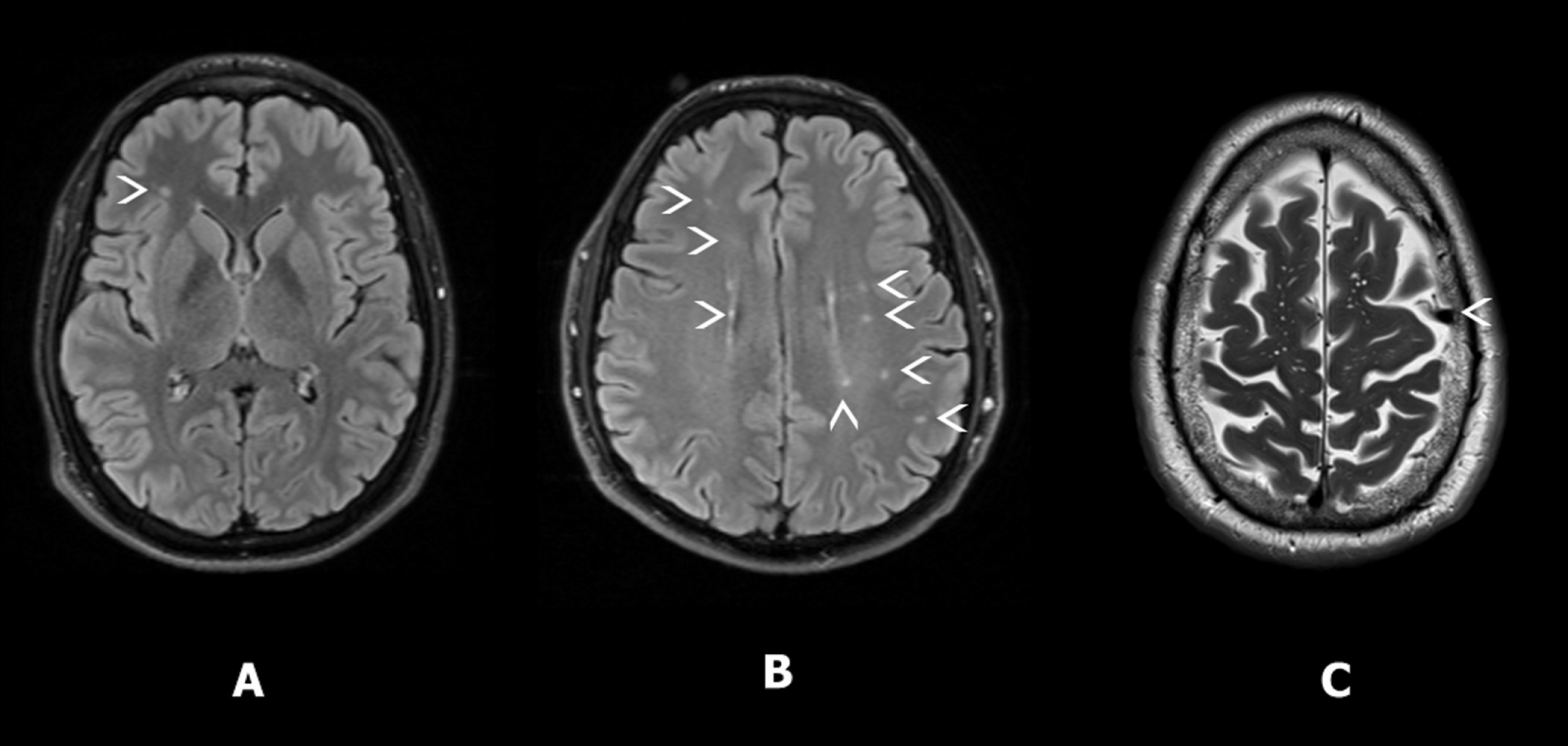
Examples of MRI incidental findings. Relevant details are marked with white arrowheads: A. Unspecific punctate lesion in a 21-year old male, in FLAIR. B. Multiple foci of white matter hyperintensity, corresponding to stage 1 on the Fazekas scale,[32] visible in the FLAIR scan in a 50-year old man. C. Venous angioma in a 58-year old man in a T2-weighted image.
